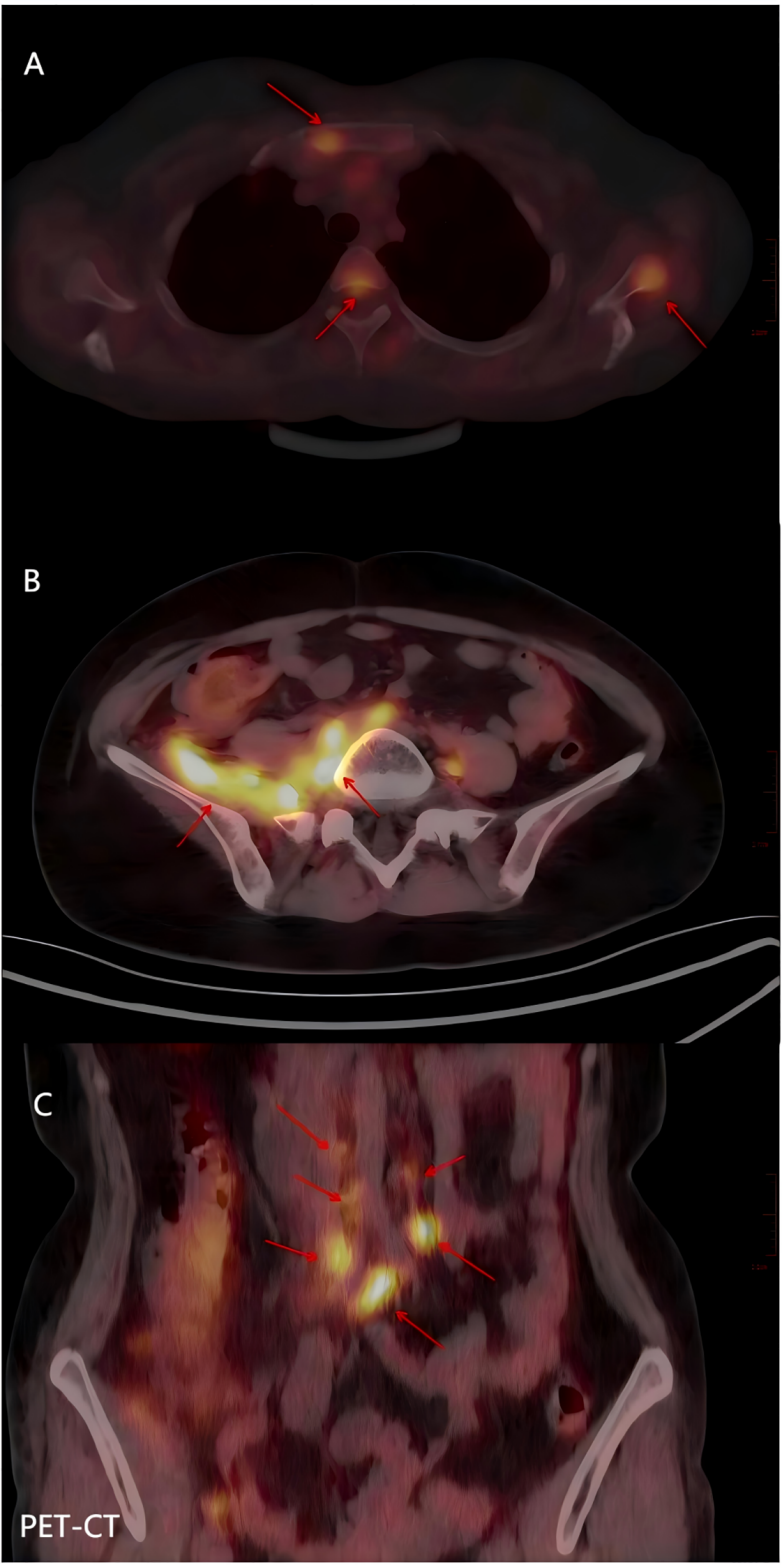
PET-CT of a patient who relapsed 8 months after completion of chemoradiotherapy (A) The sternum, thoracic vertebrae and scapula had increased FDG metabolism, and metastatic carcinoma was considered. (B) The FDG metabolism of the right pelvic wall muscle was increased, and metastatic carcinoma was considered. (C) The FDG metabolism of lymph nodes adjacent to bilateral common iliac vessels and abdominal main vessels was increased, and metastatic carcinoma was considered.
